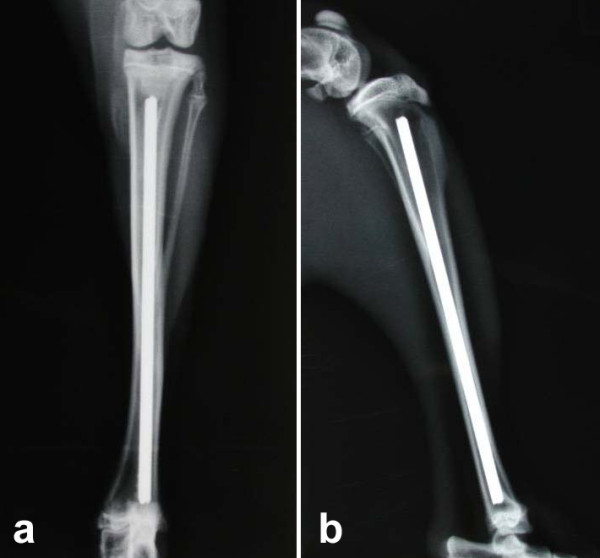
X-ray of the tibia postoperatively (a) a.p.-view (b) lateral view.
